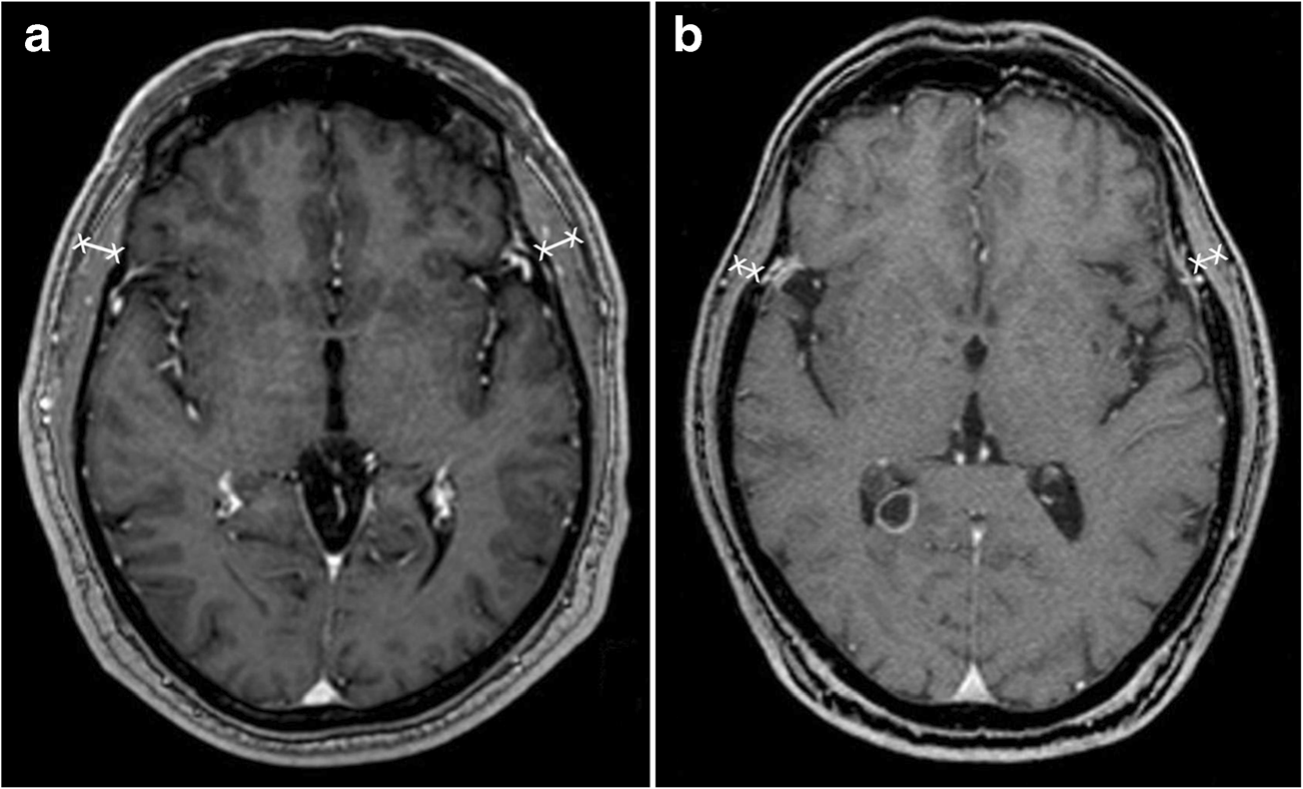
Magnetic resonance images representing temporal muscle thickness (TMT) assessment. (A) A 58-year-old male patient with an overall survival of 59 months (median TMT = 11.2 mm), and (B) a 68-year-old male patient with an overall survival of 1 month (median TMT = 4.6 mm)
